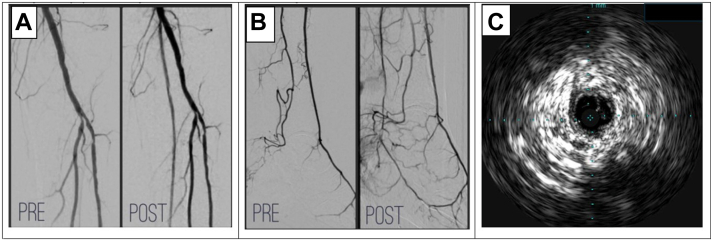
RIVER Technique for Revascularization of an Anomalous Posterior Tibial Artery (A) Pre- and postrevascularization of anomalous posterior tibial artery. (B) Pre- and postrevascularization of dorsal pedal loop. (C) Intravascular ultrasound image showing intraluminal access of anomalous posterior tibial artery.
